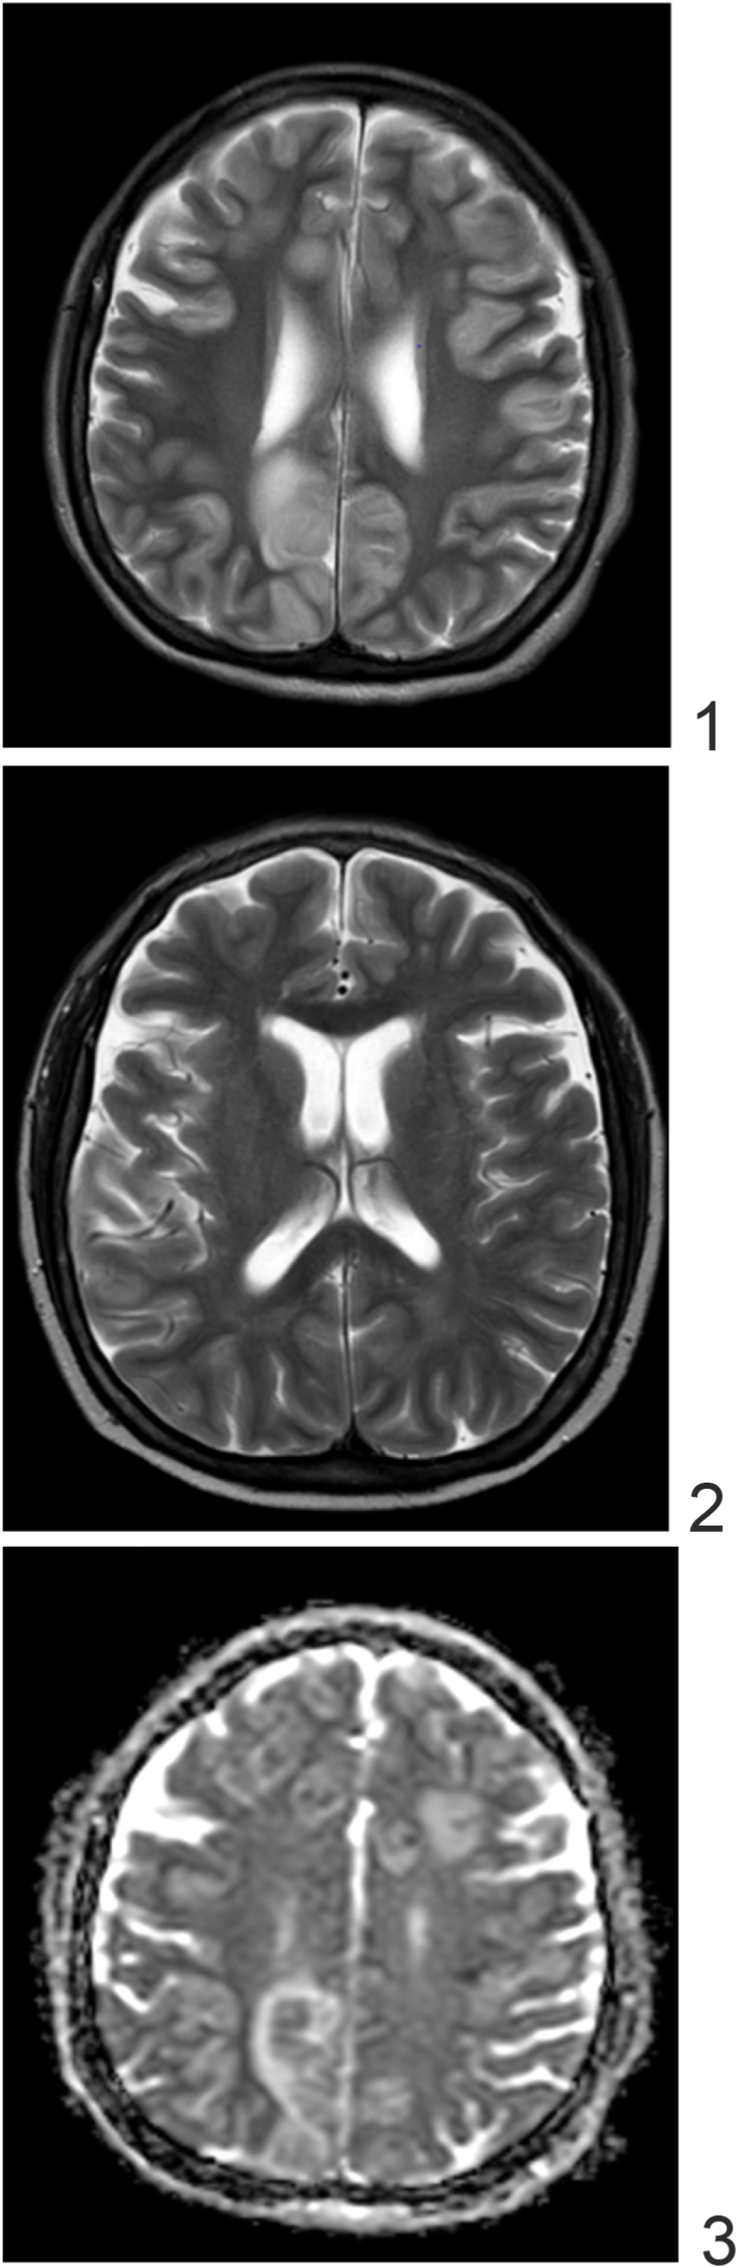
MRI brain with contrast: Figs. 1–3: Extensive abnormal T2 hyperintense signal in the cerebral cortex compatible with meningoencephalitis.
